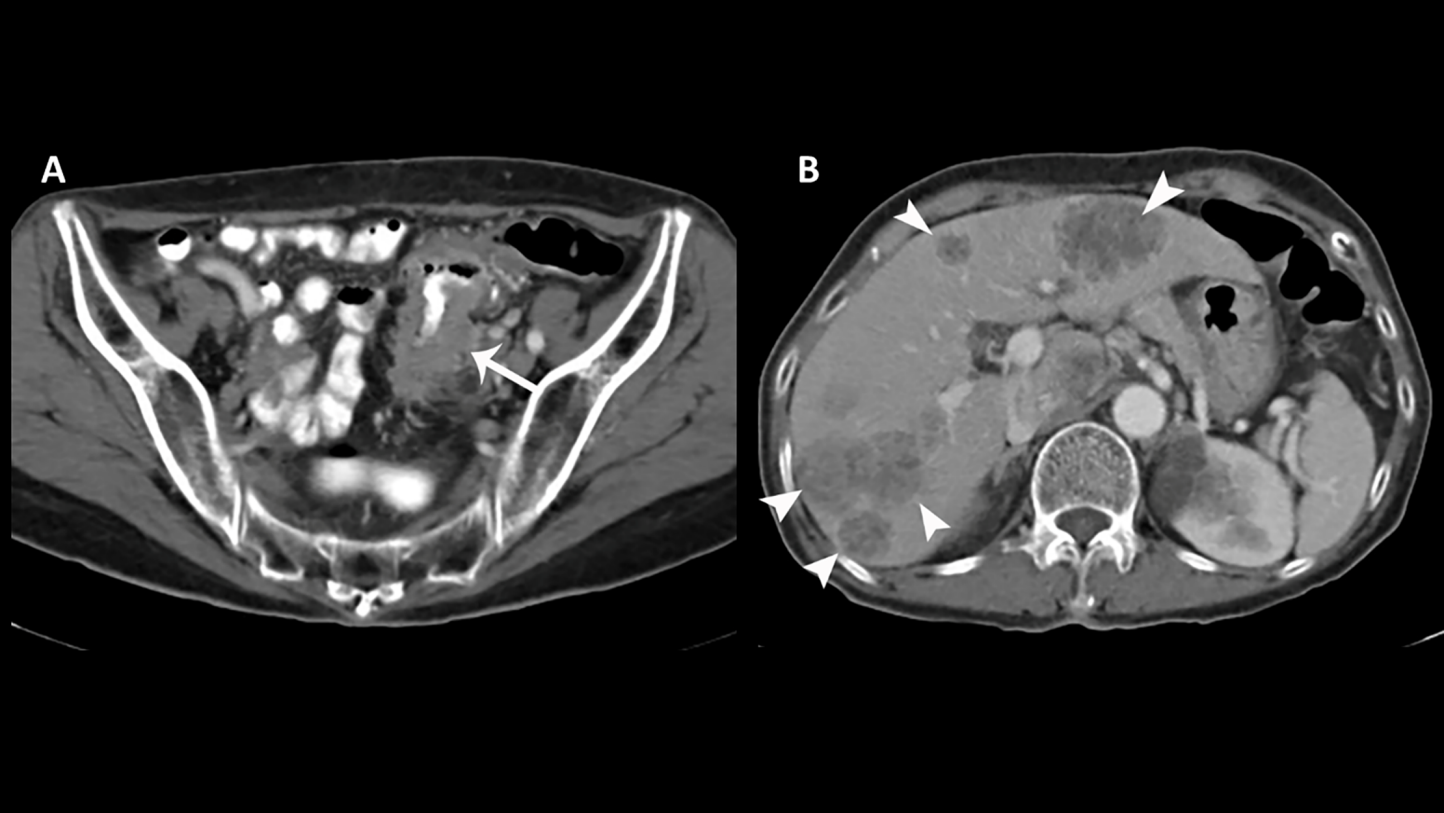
True positive category 1 scan—findings highly suspicious for organic etiology. (A) Axial CT scan of an 80 year old male demonstrates bowel wall thickening of the sigmoid colon (arrow) suspicious for underlying malignancy. (B) Multiple hypodense hepatic lesions are consistent with metastases (arrowheads).
